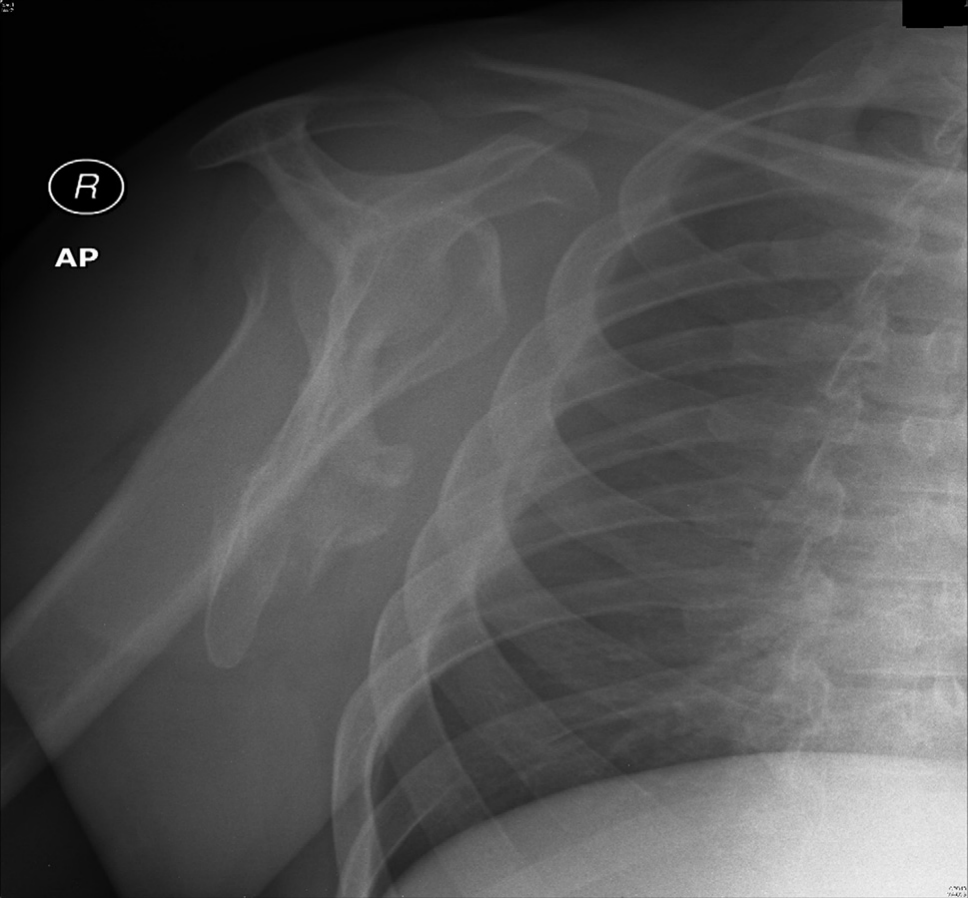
Lateral view of plain radiograph of right scapula demonstrating a broad based pedunculated osteochondroma.
